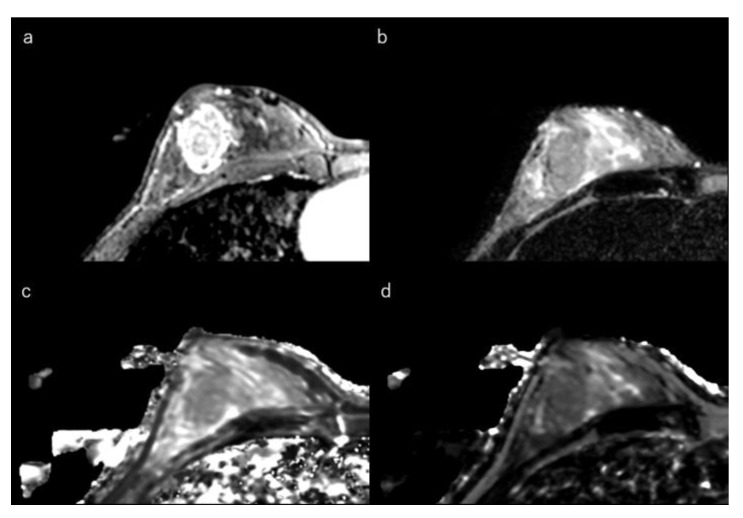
(a) Dynamic contrast-enhanced MR image in a 32-year-old woman with luminal-B type breast cancer shows a heterogeneously enhanced oval mass with rim enhancement. (b) Short-tau inversion recovery image shows an intermediate signal mass. (c) T1 map (window width/centre = 1400/2400 ms) shows an intermediate signal mass; Mean T1 of the mass is 1174 ms on T1 map. (d) T2 map (window width/centre = 160/240 ms) shows an intermediate signal mass; mean T2 of the mass is 97 ms on T2 map.
